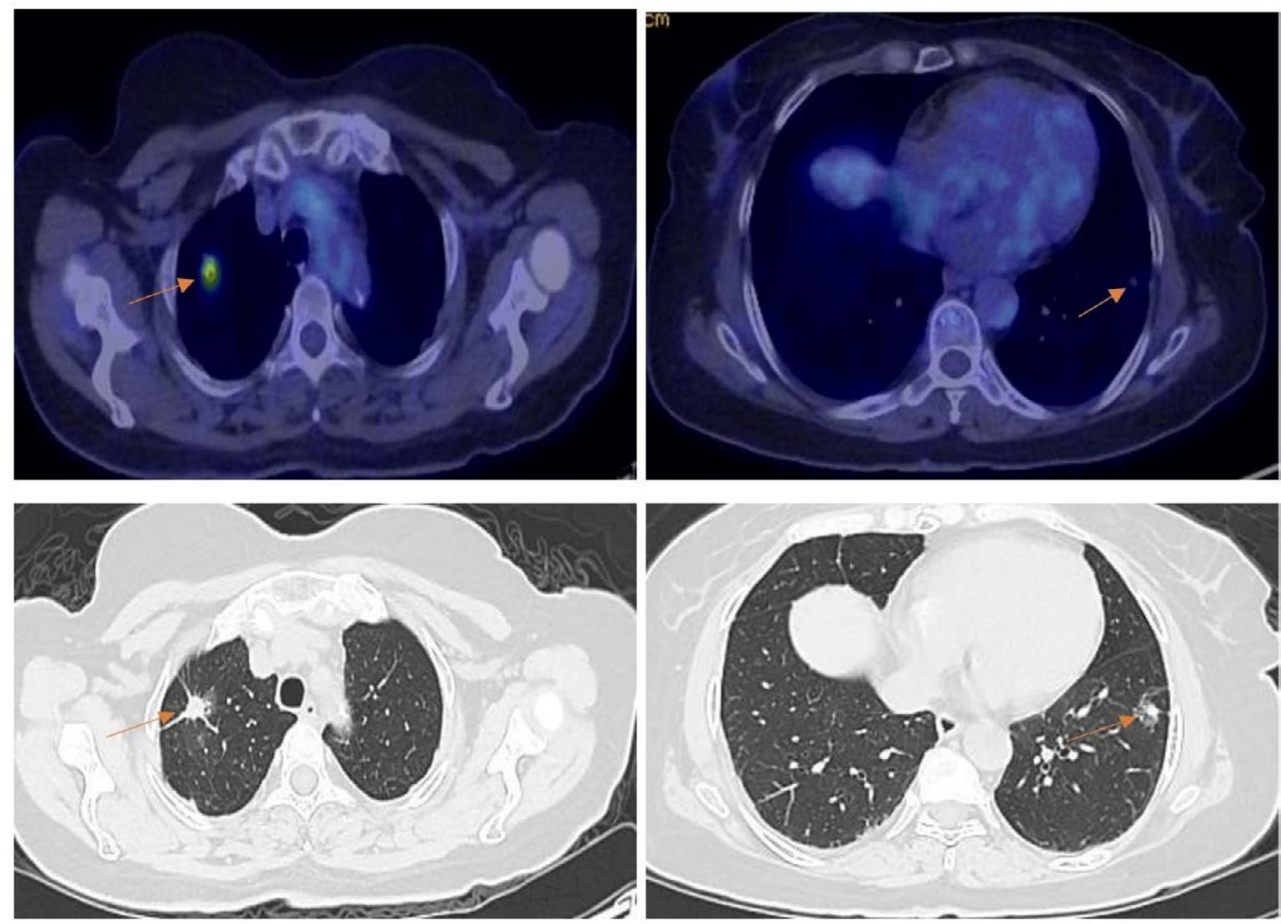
PET‐CT of bilateral primary lung masses. PET‐CT revealed a spiculated solid nodule in the right upper lobe with increased FDG uptake (SUVmax: 7.3), measuring 15 mm in diameter, and a part‐solid nodule with irregular borders was detected in the left lower lobe, measuring 15.3 mm with mildly increased FDG uptake (SUVmax: 1.3).
